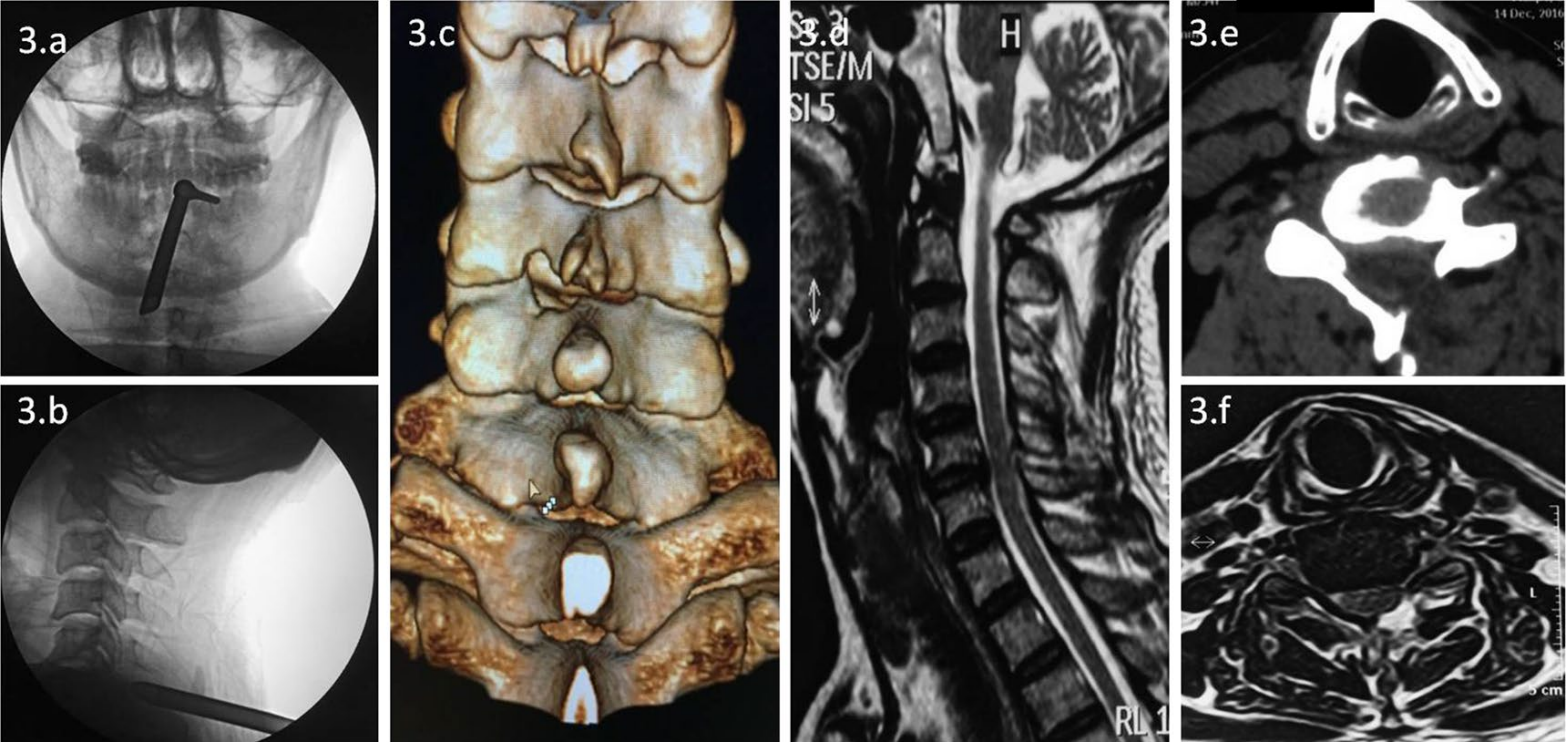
Intraoperative fluoroscopy and postoperative images of the key-hole technique. Intraoperative fluoroscopy confirmed that the working channel was located in the lesion segment (a, b). The 3D reconstruction image (c) shows the bone structure removed by the key-hole technique. Sagittal and axial MRI (d, f) showed no herniation of the spinal cord or nerve. Axial CT (e) showed no disc herniation compressing the spinal cord or nerves.
